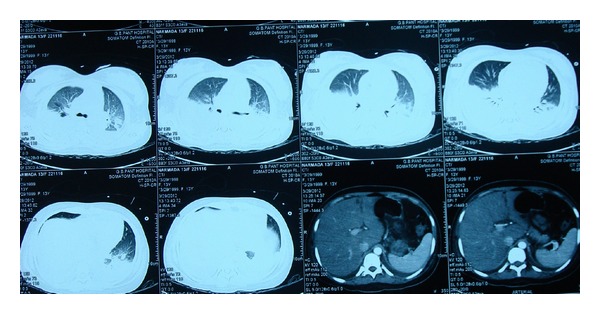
CT sections showing bilateral pleural effusion with atelectatic lower zones.
